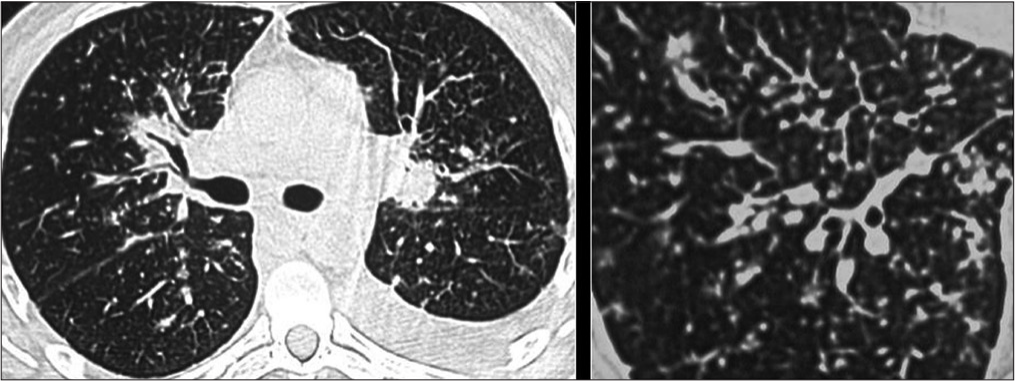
Kaposi’s sarcoma. High-resolution computed tomography shows thickening of the bronchovascular bundles reflecting bronchocentric disease. Interlobular septal thickening due to lymphatic obstruction because of tumor invasion is also seen
